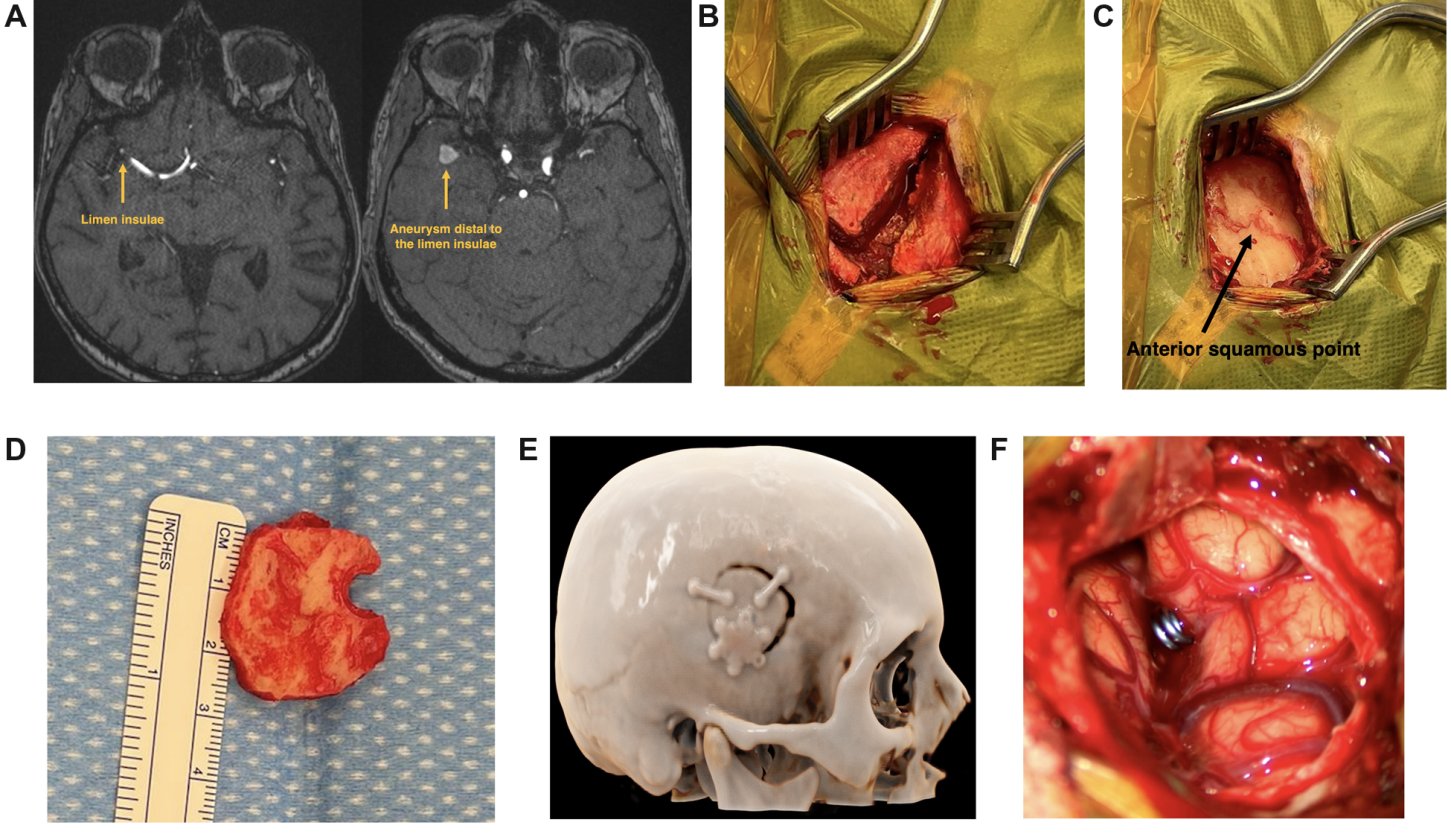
Sylvian keyhole approach case. (A) Native time of flight angiography MRI with a right-sided MCA aneurysm, located distally to the limen insulae. (B) Temporal muscle incision (T-shape incision). (C) The craniotomy is typically centered around the anterior squamous point. (D) 2.5 cm × 2.5 cm sized craniotomy. (E) Cinematic rendering of the postoperative CT-scan, showing location and size of the SKA. (F) Focal opening of the sylvian fissure (1.5 cm).
